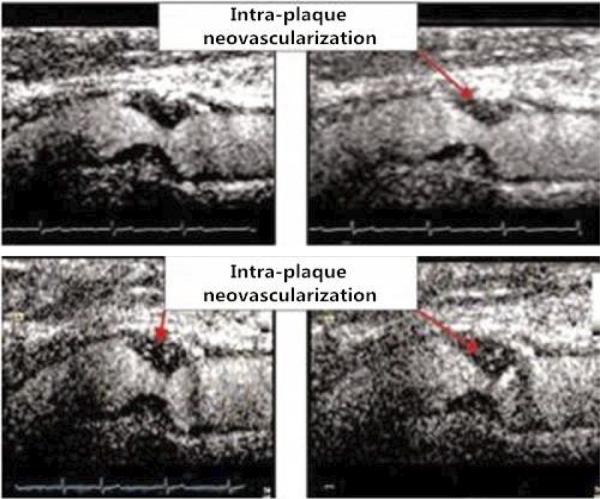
A sequence of images obtained following the intravenous injection of ultrasound contrast indicates the presence of intra-plaque neovascularization in the sequential frames (Grade 2.5, extensive neovascularization). The presence and degree of neovascularization were determined by surgery and laboratory testing.
